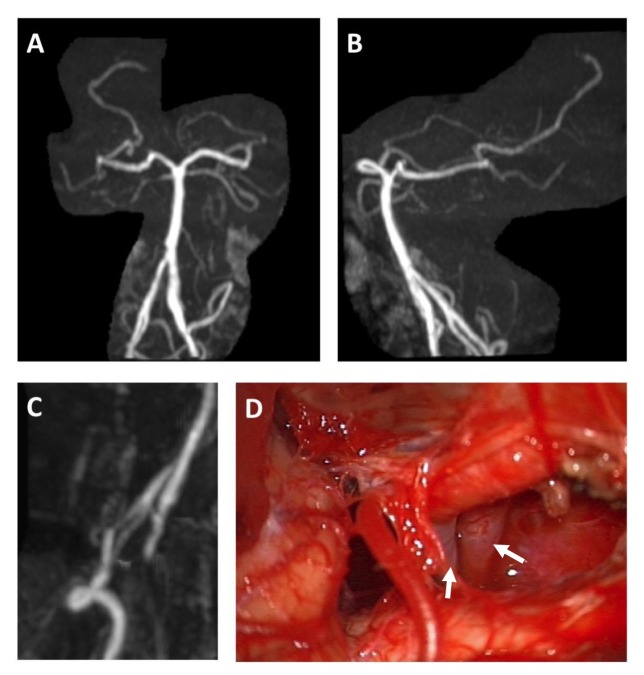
Radiographic and Intraoperative Images of Fenestrated Vertebral Artery MRA time of flight study demonstrating rotated views of the vertebrobasilar circulation. The right vertebral artery demonstrates fenestration in the V4 segment (A-C). Intraoperative photo demonstrating intradural origin and fenestration of the right vertebral artery (D).
